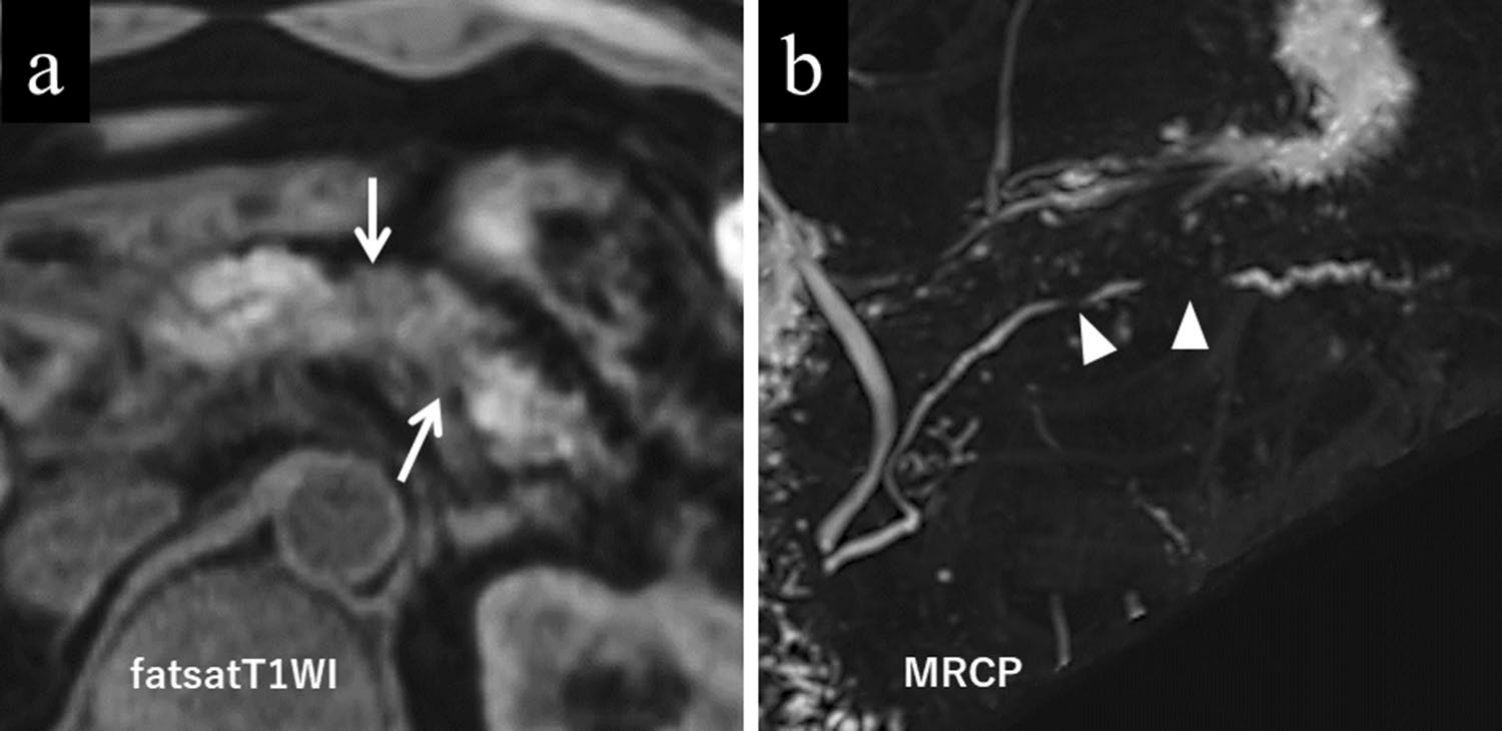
Typical MRI images seen in a male patient in his 50 s with autoimmune pancreatitis. a The lesion is hypointense on fat-saturated T1-weighted image (arrows). b Multiple stenotic segments of the main pancreatic duct (skip narrowing and the icicle sign) are seen (arrowheads) on MRCP
